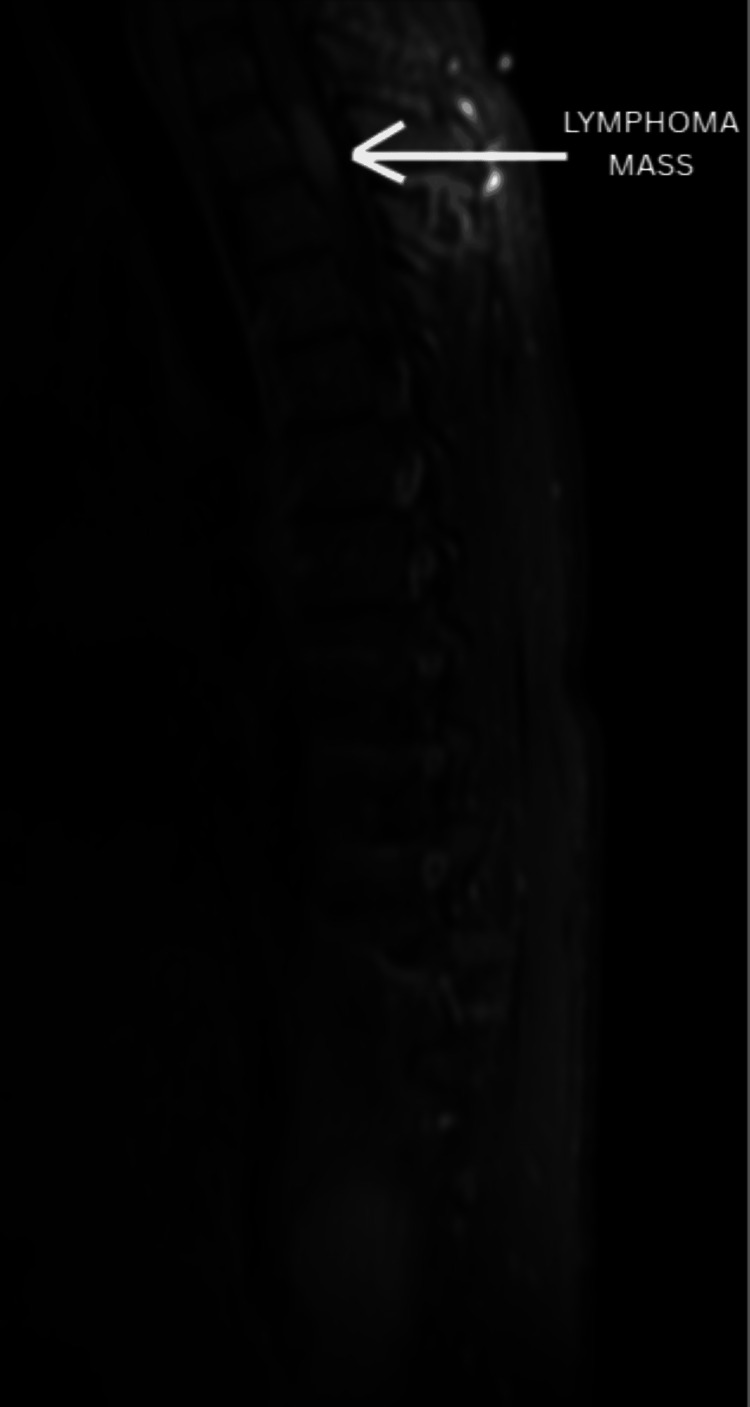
Sagittal T2-weighted MRI Sagittal T2-weighted MRI demonstrates an expansile intramedullary lesion centered at T2-T3 (arrow), in the proximal thoracic cord, measuring approximately 2.3 cm in length and involving nearly the entire cord at this level. Associated T2 hyperintensity extends above and below the lesion. Abbreviation: MRI, magnetic resonance imaging.
